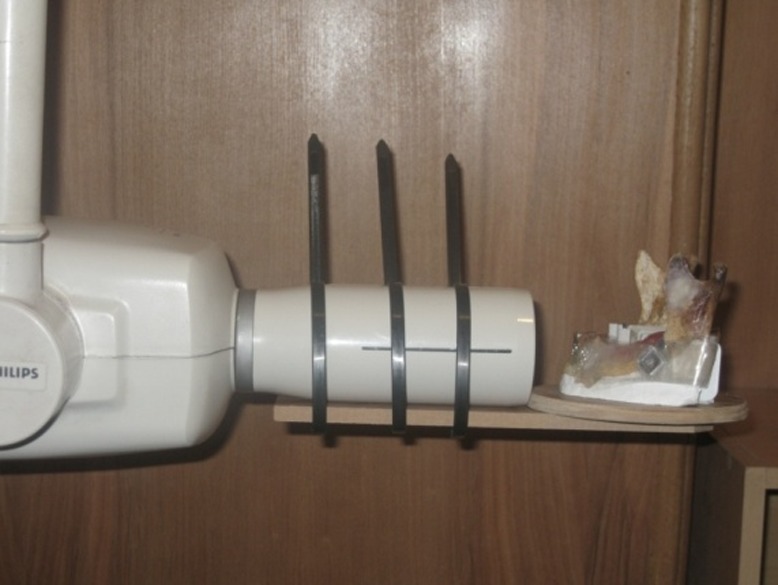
The radiographs were taken with an intraoral radiographic machine (ORALIX 65S, Philips, Italy) in the Radiology Department, Faculty of Dentistry, Tabriz University of Medical Sciences. Square (10 mm × 10 mm) and triangular (13 mm × 10 mm ×10 mm) aluminum plates with a thickness of 33±2 μ were provided. E-speed films (Kodak, France) were used to take radiographs from the right and left molar and anterior regions of the mandibles using film holders and the mandible holding device in paralleling technique under the same radiographic condition (mA=7.5, kVp=65, time=0.8 seconds). At first a periapical radiograph and a direct digital radiograph were taken in the anterior region without placing aluminum plates; Kodak RVG (5100, UK) was used for the direct digital technique.
